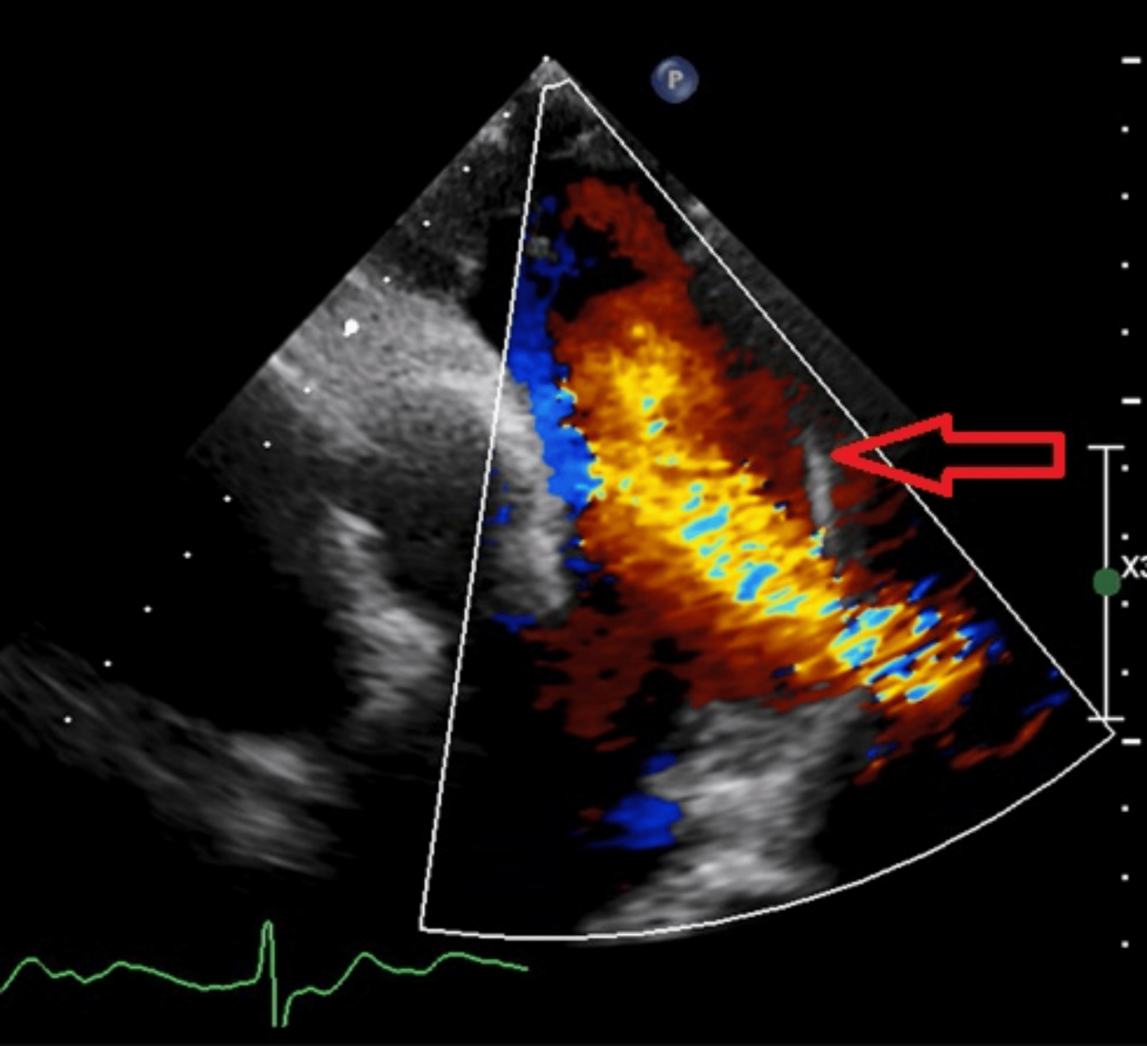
Transthoracic echocardiogram showing a PDA (arrow) PDA: patent ductus arteriosus
